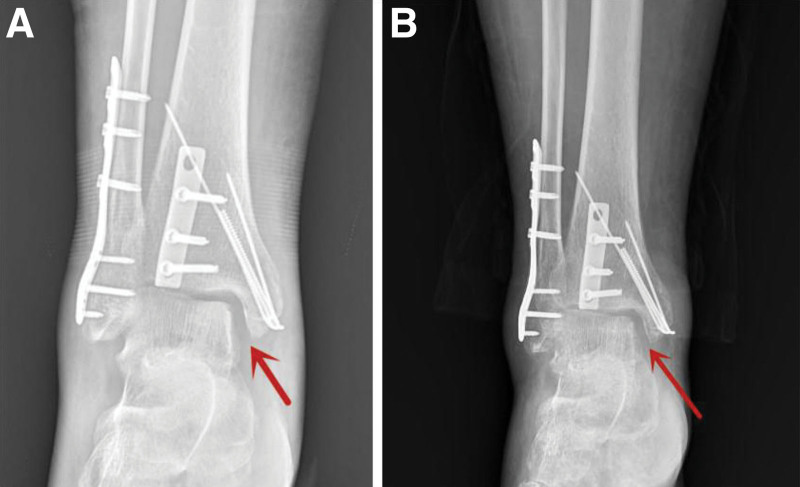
X-ray imaging in his periodic review. (A) Two months after surgery; (B) five months after surgery. The red arrows are pointing to the widen medial articular space.
